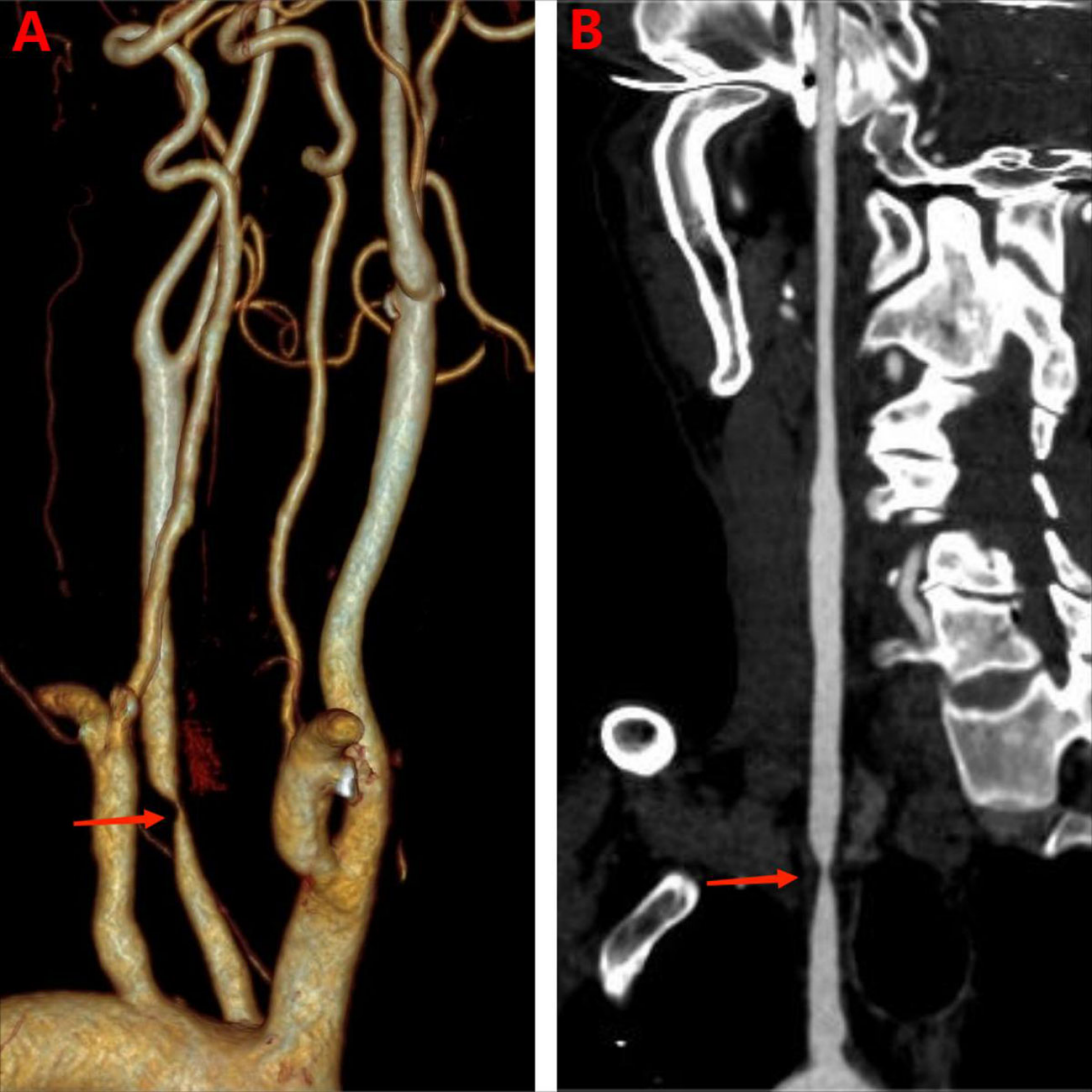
CTA imaging. (A) Three-dimensional reconstruction images of the patient before treatment. The arrow indicates severe stenosis of the left internal carotid artery. (B) Pre-treatment tomographic images of the patient. The arrows indicate severe stenosis of the left internal carotid artery.
